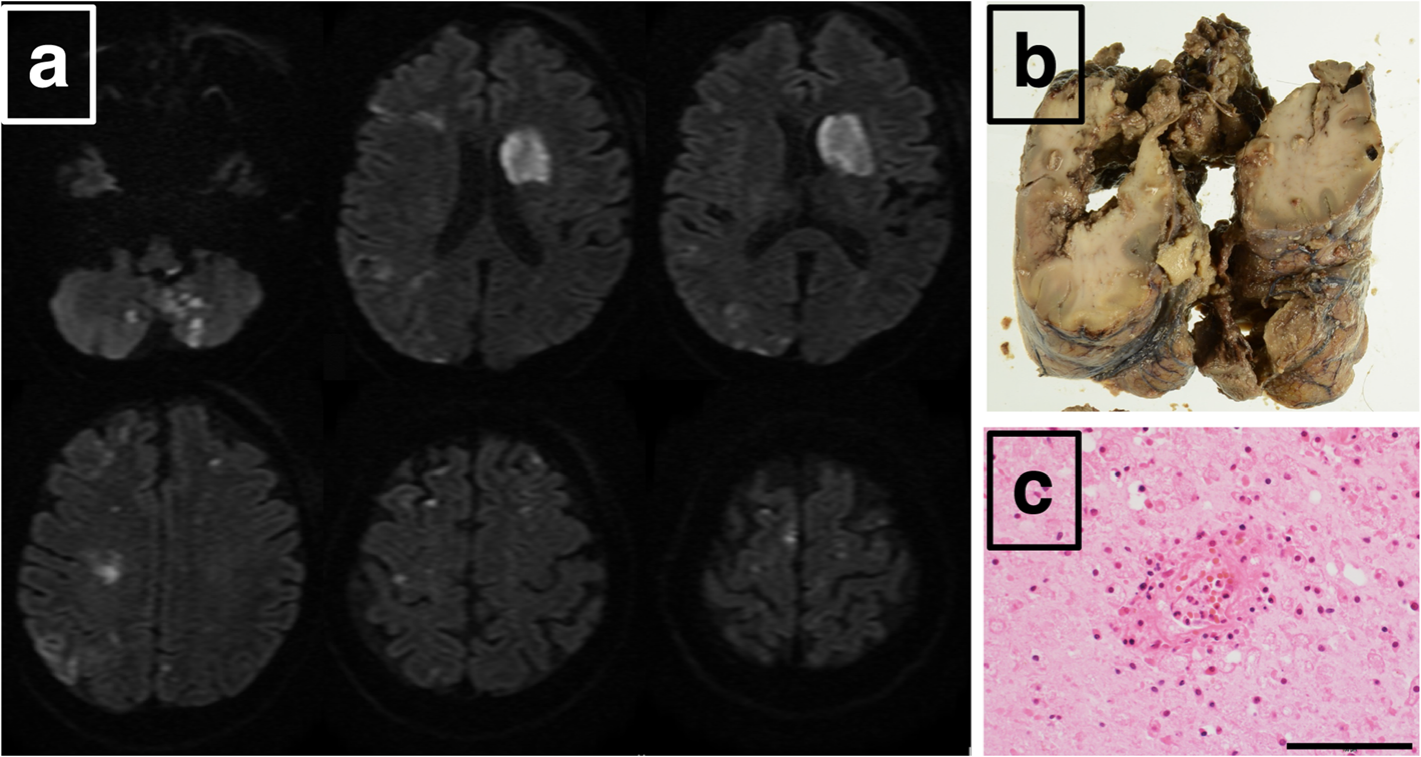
Diffusion Weighted Magnetic Resonance Imaging of cerebrum (a) / brain autopsy findings (b) / Eosinophilic granulomatosis in brain (c). On admission, there were multiple infarctions which had a mixture of small infarction and large hemorrhagic infarction. Multiple small cerebral infarct lesions in the cortex and subcortex were caused by vasculitis and eosinophil infiltration
